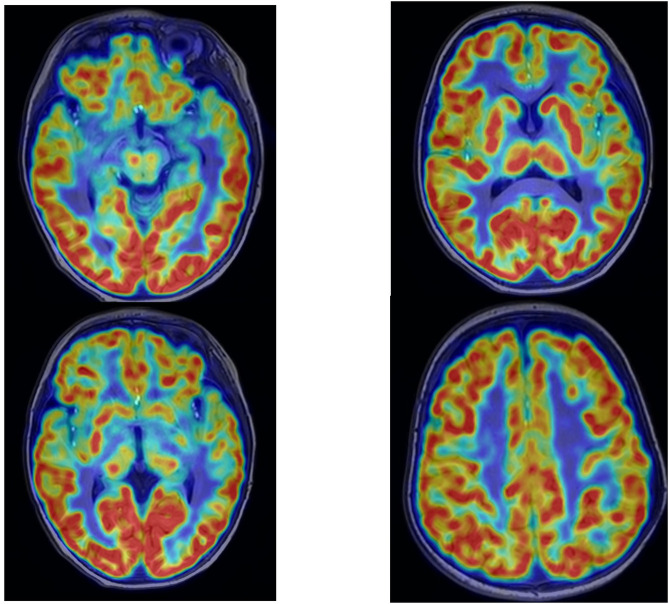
Cranial 18F-FDG PET-MRI fusion: mild hypometabolism in the medial left frontal lobe without significant epileptogenic hypometabolic foci.
